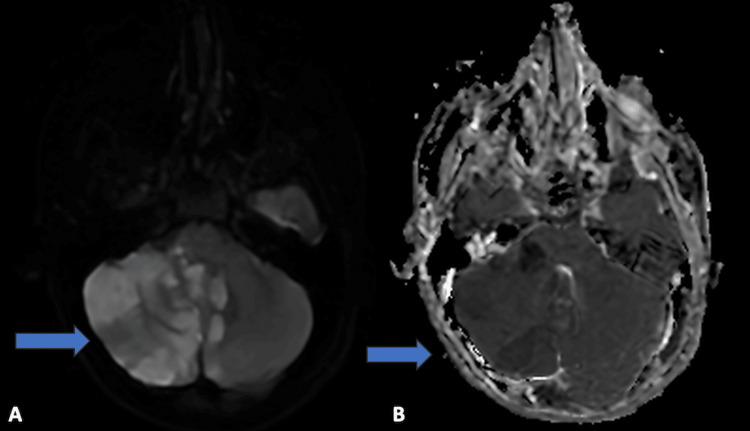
Restricted diffusion on diffusion-weighted imaging (DWI) (A), with corresponding low apparent diffusion coefficient (ADC) signal (B) in the right cerebellar hemisphere, consistent with a focal infarct.
